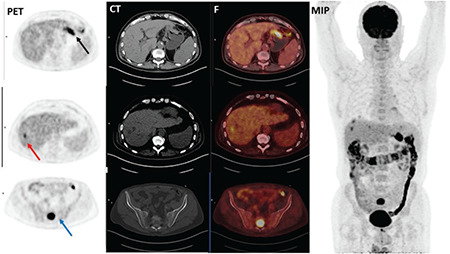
A 68-year old male patient with gastric tubular adenocarcinoma. Axial PET (A), CT (B), and fusion (C) images showed high ^18^F-FDG uptake (SUV_max_: 13.29) in primary tumor in the fundus of the stomach (black arrow). Liver metastasis showed increased ^18^F-FDG uptake (short axis diameter: 1.88 cm, SUV_max_: 6.24) (red arrow). Additionally, bone metastasis was demonstrated in PET/CT images (SUV_max_:16.29) (blue arrow) ^18^F-FDG: Fluorine-18-fluorodeoxyglucose, SUV_max_: Maximum standardized uptake value, MIP: Maximum intensity projection image, PET: Positron emission tomography, CT: Computed tomography
